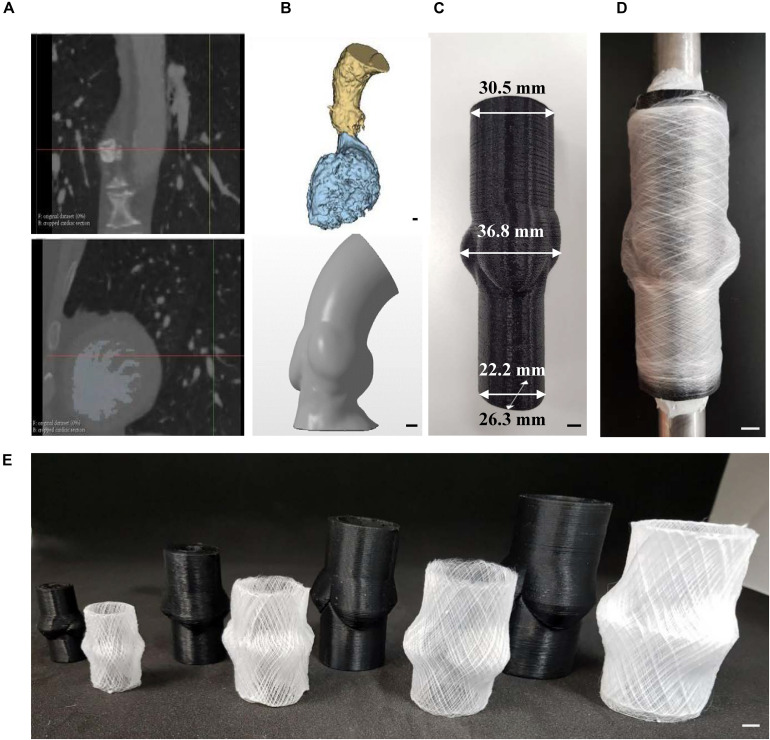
Fabrication of personalized aortic root scaffolds. (A) CT scans of the patient’s aorta. (B) DICOM to STL conversion using InVesalius software and smooth model of the personalized aorta. (C) PLA 3D printed model of the personalized aorta to be used as the substrate mandrel for MEW. (D) MEW performed on the 3D printed personalized mandrel. (E) 3D printed mandrel models and corresponding MEW scaffolds of 10, 15, 20, and 25 mm diameter. (Scale bar: 5 mm).
